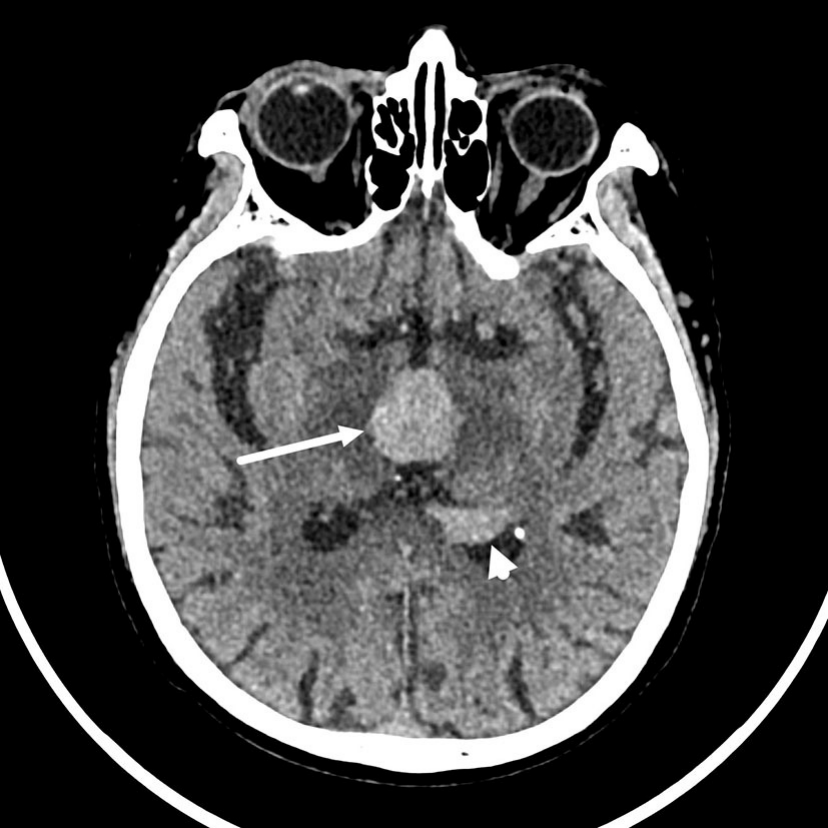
Non-contrast computed tomography (CT) shows that the masses with slight hyper-attenuation were in the third ventricle (the tumor size: 22 mm × 22 mm × 15 mm, CT value: 47HU) (arrow), in the body (not shown) and posterior horn (arrowhead) of the left lateral ventricle.
